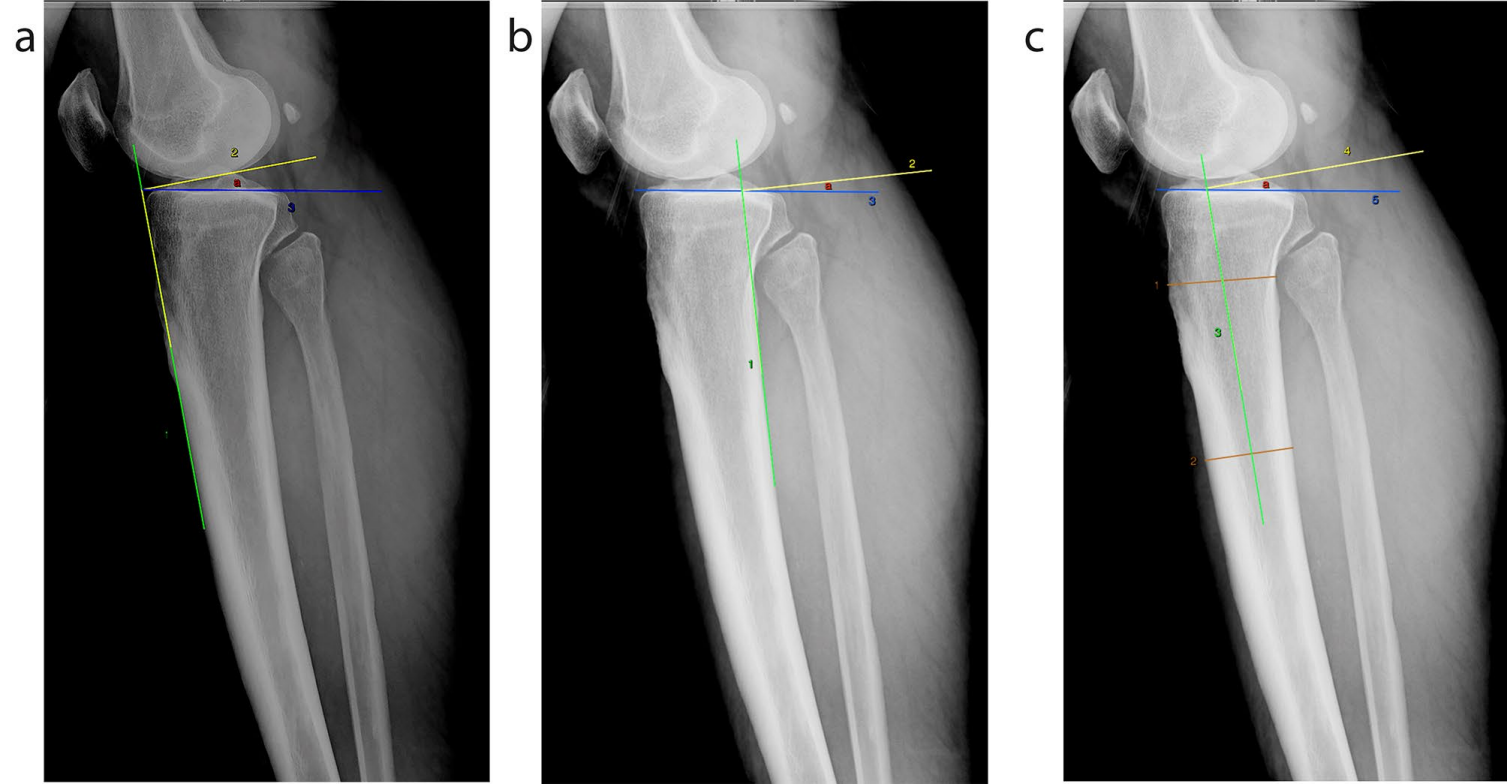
a-c: Posterior tibial slope measurement methods in left knee lateral radiograph. Anterior tibial cortex (a), posterior tibial cortex (b), and proximal tibial anatomical axis (c)
